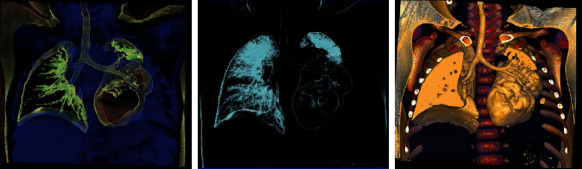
Noncontrast frontal view chest CT, image reformatting volume rendered display: coronal reformation in standard lung window to allow three-dimensional view. Pneumatoceles, thin-walled cavities developing secondary to necrosis.
